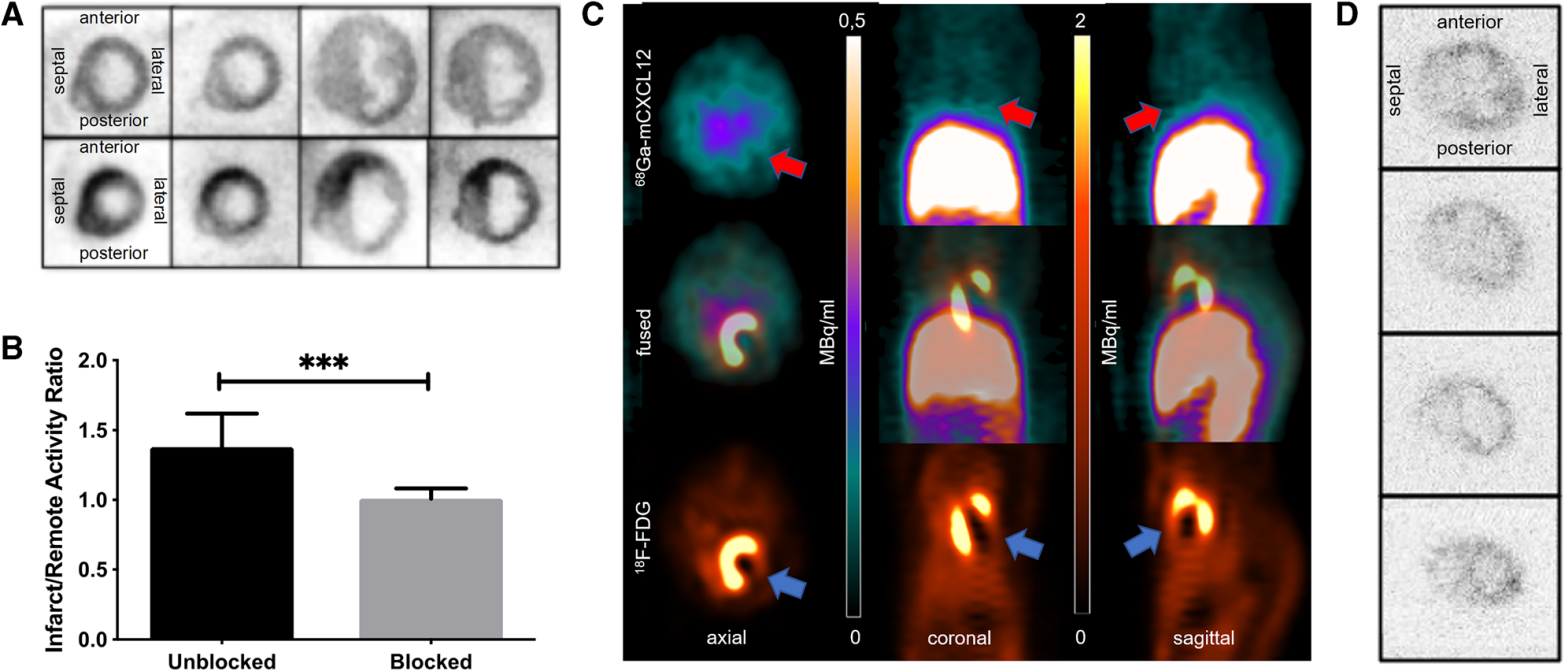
Specificity of ^68^Ga-mCXCL12. (A) Unblocked slices with ^68^Ga-mCXCL12 accumulation in the infarcted heart in the *anterior* and *antero-septal* wall (bottom row). After blocking with CXCR4 antibody sections show no visible ^68^Ga-mCXCL12 uptake anymore (top row). (B) Significantly higher ^68^Ga-mCXCL12 myocardial infarct-to-remote activity ratios as compared to the unblocked slices. All data represent mean ± SD. (C) PET image on day 2 shows no visible ^68^Ga-mCXCL12 uptake in the infarcted myocardium after Co-Injection with excess of native mCXCL12. ^18^F-FDG PET showing the viable myocardium (red arrow: no ^68^Ga-mCXCL12 uptake; blue arrow: infarcted myocardium). (D) Autoradiography on day 2 after Co-Injection with excess of native mCXCL12 shows no significant ^68^Ga-mCXCL12 uptake (top to bottom shows representative slides from the base to the apex of the heart)
